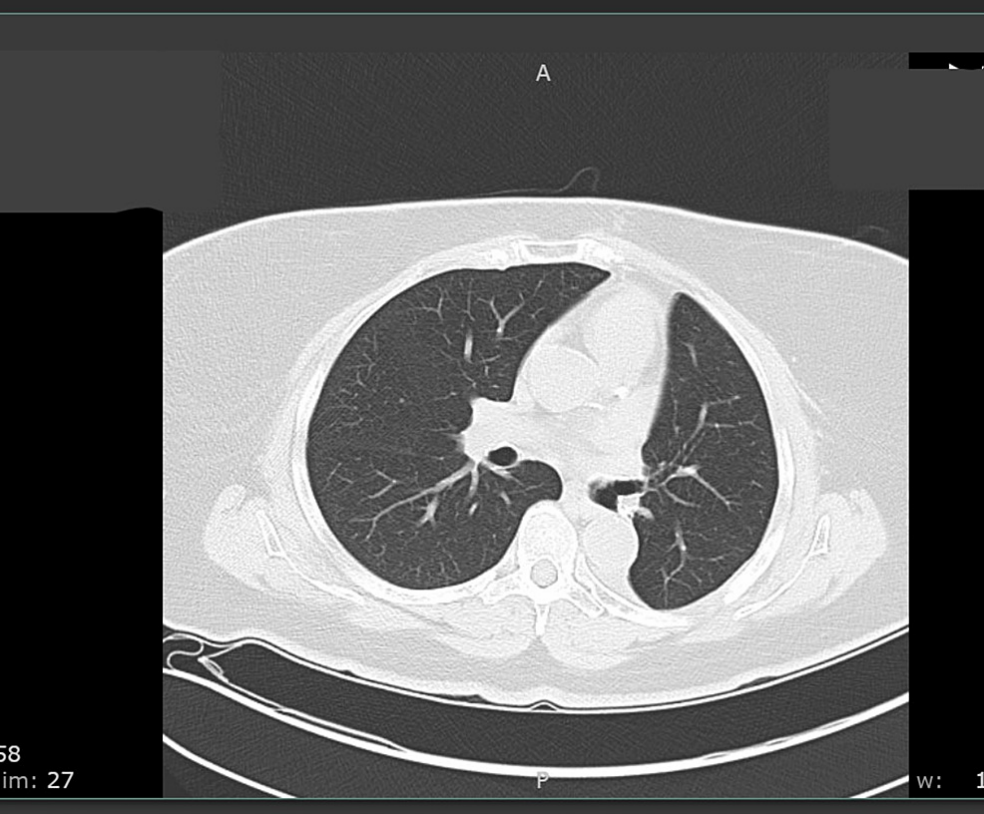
Five Month Post-Operation Axial CT Chest Without Contrast CT, computed tomography
